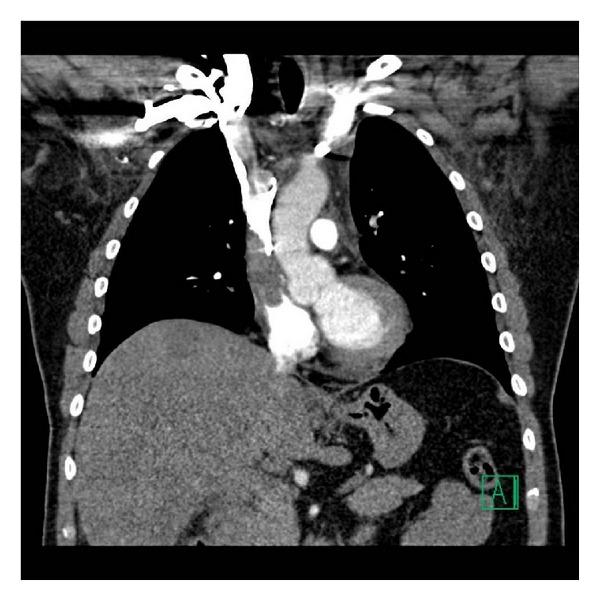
CT scan showing a thrombus extending from the internal jugular vein to the right atrium.
